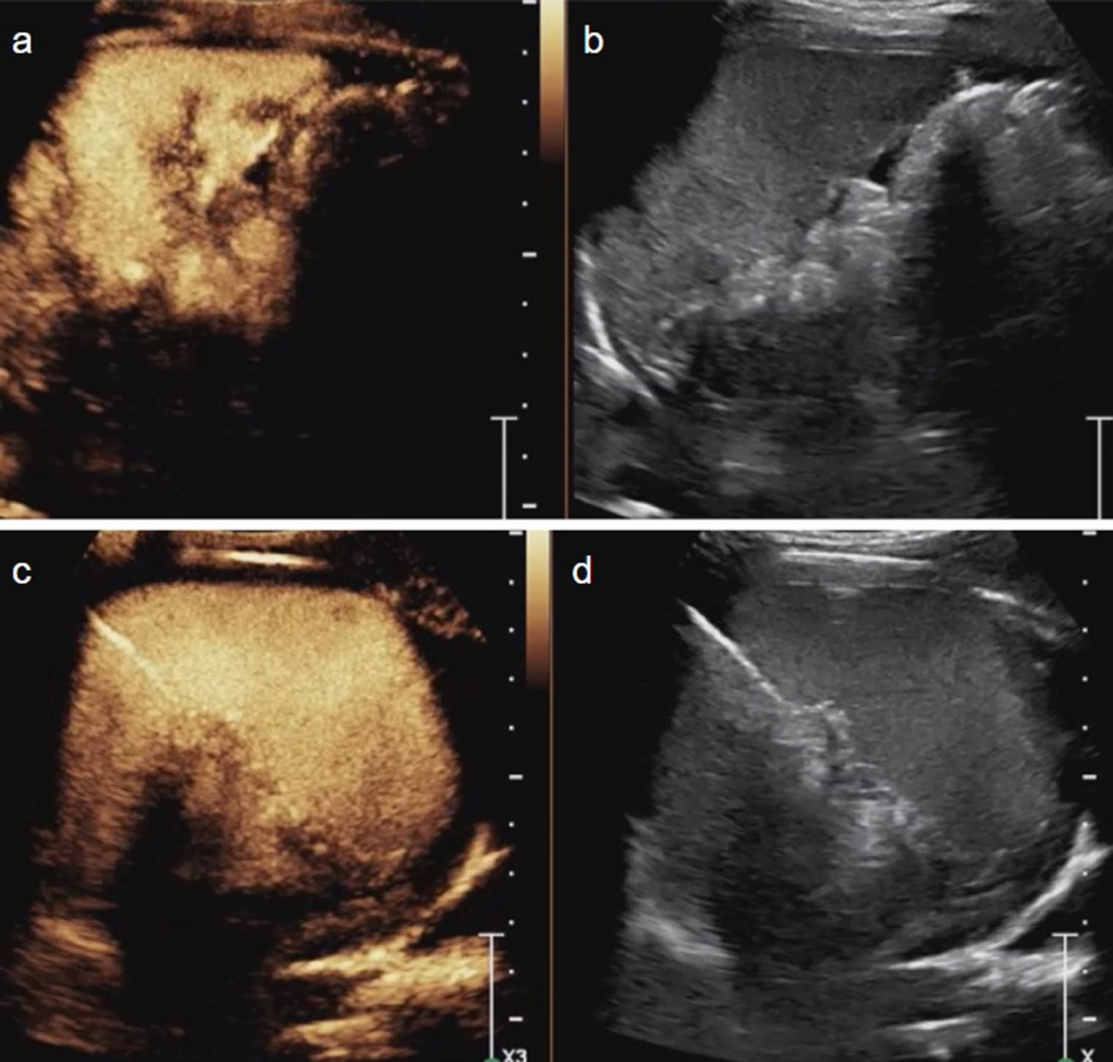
Normal spleen in a pediatric patient. (a-d) Transverse contrast-enhanced ultrasound (CEUS) images of the spleen with a dual display of contrast (left) and gray-scale (right) modes at 15 s (a, b) and 80 s (c, d) post contrast injection. The spleen reveals arciform enhancement in the arterial phase and homogeneous enhancement in the venous phase in the CEUS images
